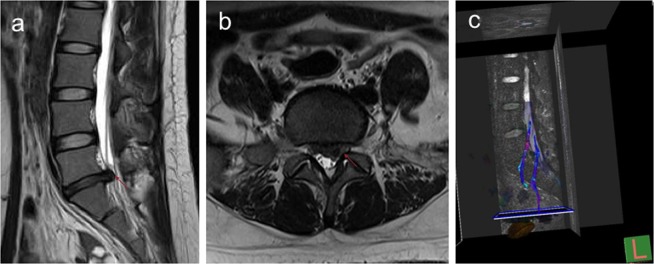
Preoperative lumbar disc MRI and lumbosacral nerve root DTI scan. (a) is the sagittal position of the patient’s preoperative MRI. (b) is the axial position of the patient’s preoperative MRI, and the protruding intervertebral disc is the left area II. (c) is the nerve root reconstructed by DTI before surgery, indicating obvious changes in the microstructure of the compressed nerve root.
